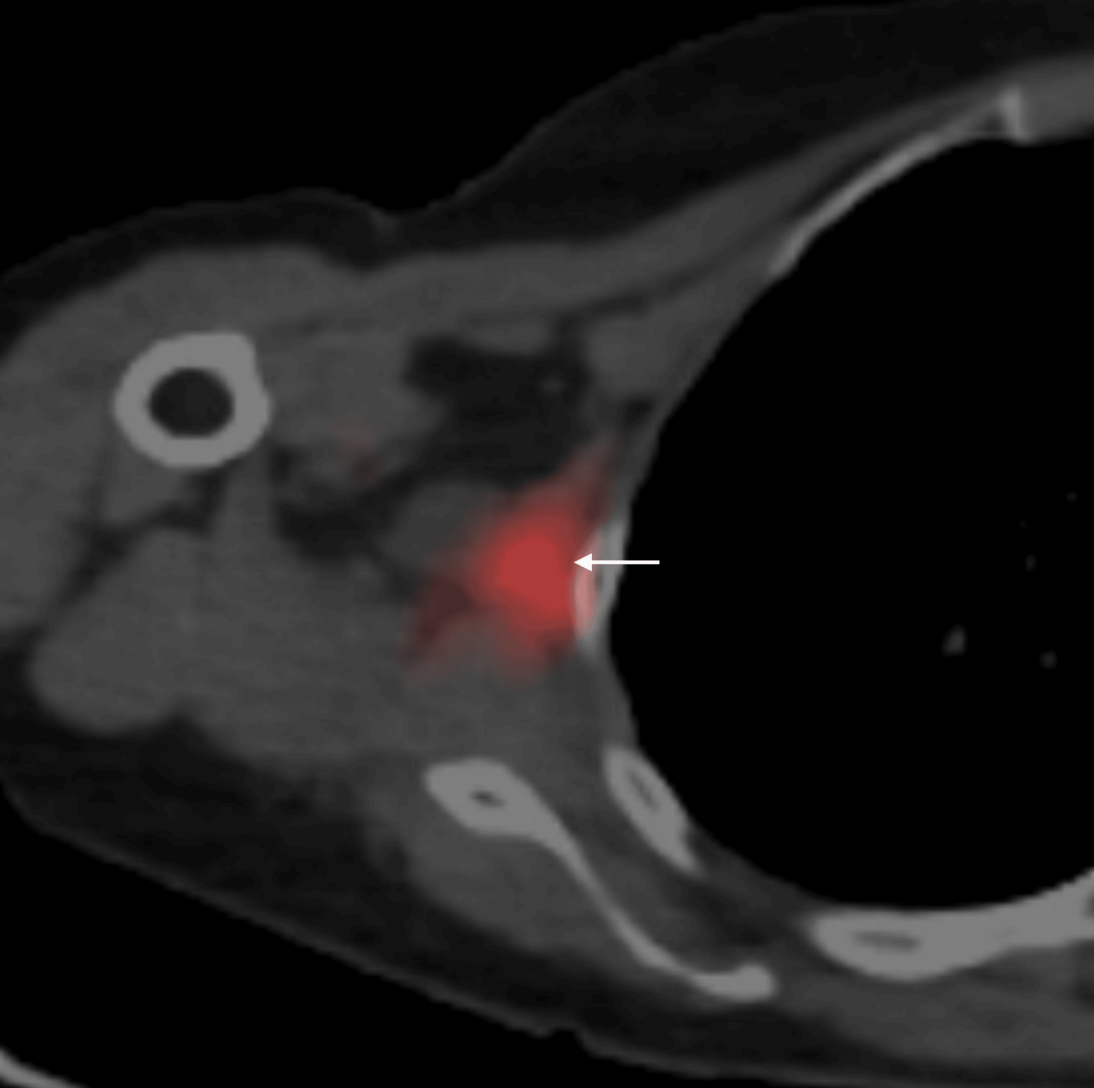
PET/CT findings PET/CT of the target mass showed a maximal standardized uptake value of 3.1 (arrow). Details of the PET examination were as follows: body weight: 59.6 kg, blood sugar: 85 mg/dL, fluorodeoxyglucose (FDG) dose: 182.6MBq, start of PET examination: 60 minutes after FDG administration, scanner protocol 1 view/2 minutes PET/CT: positron emission tomography/computed tomography
